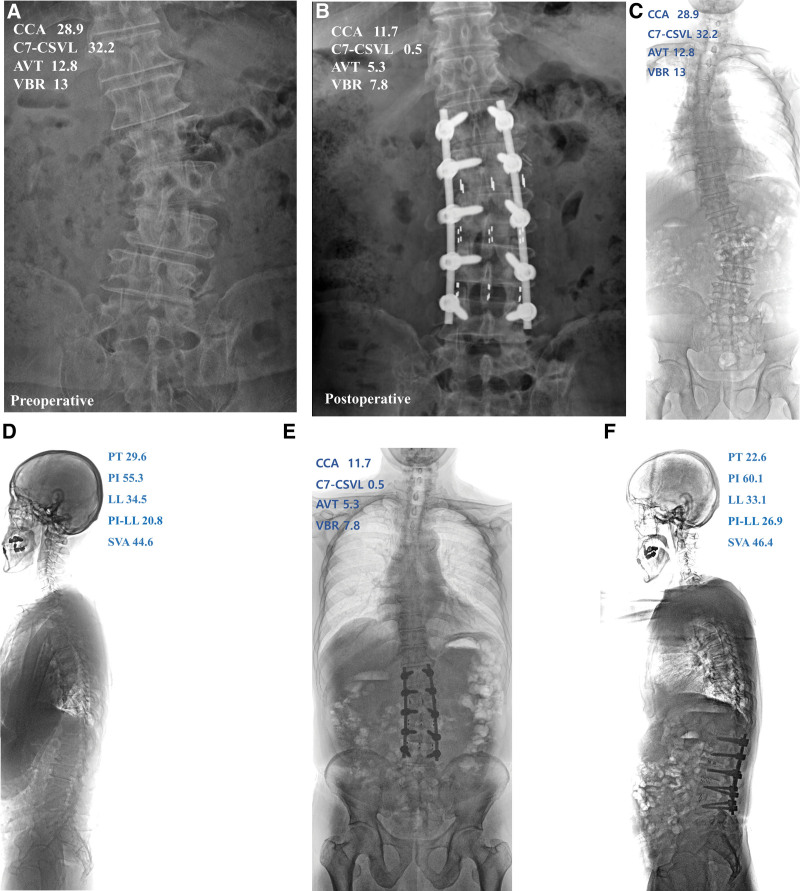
70-year-old male presented with increasing back pain and radiating pain on both leg. A: preoperative anteroposterior (AP) radiograph, B: postoperative AP radiographs at POD 12 months, C and D: AP and lateral view of preoperative standing full-length spinal radiograph, E and F: AP and lateral view of postoperative standing full-length spinal radiograph. CCA: coronal Cobb angle. C7-CSVL: distance from C7 plumb line to central sacral vertical line, AVT = apical vertebral translation, LL = lumbar lordosis, PT = pelvic tilt, PI = pelvic incidence, POD = postoperative day, SVA = sagittal vertical axis, VBR = vertebral body rotation.
